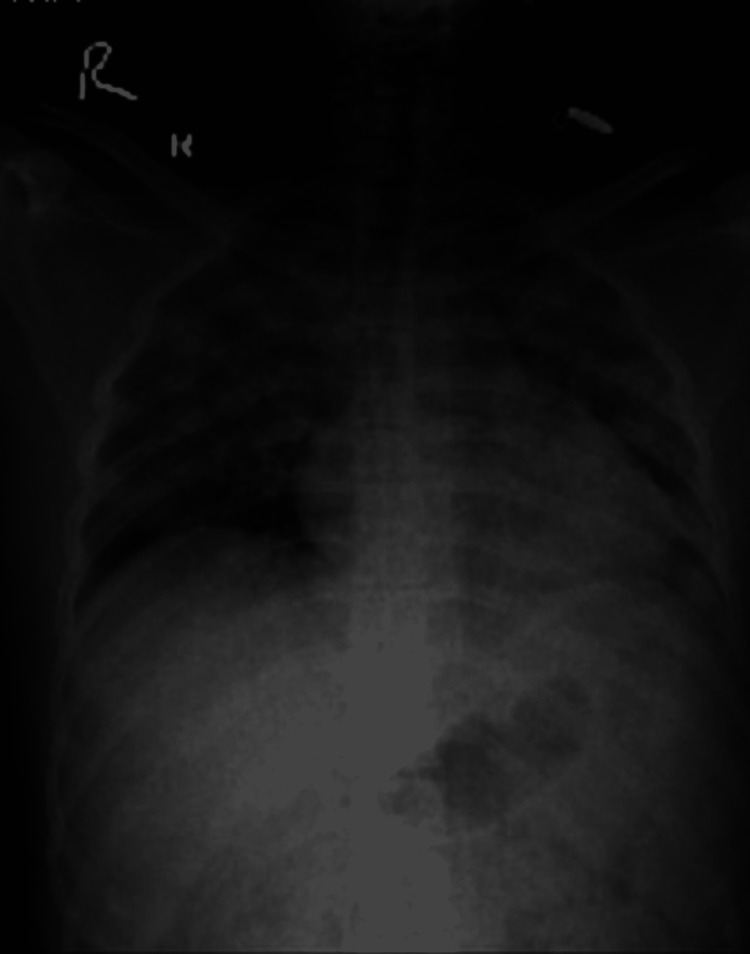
Chest X-ray showing bilateral infiltrates in lung fields
